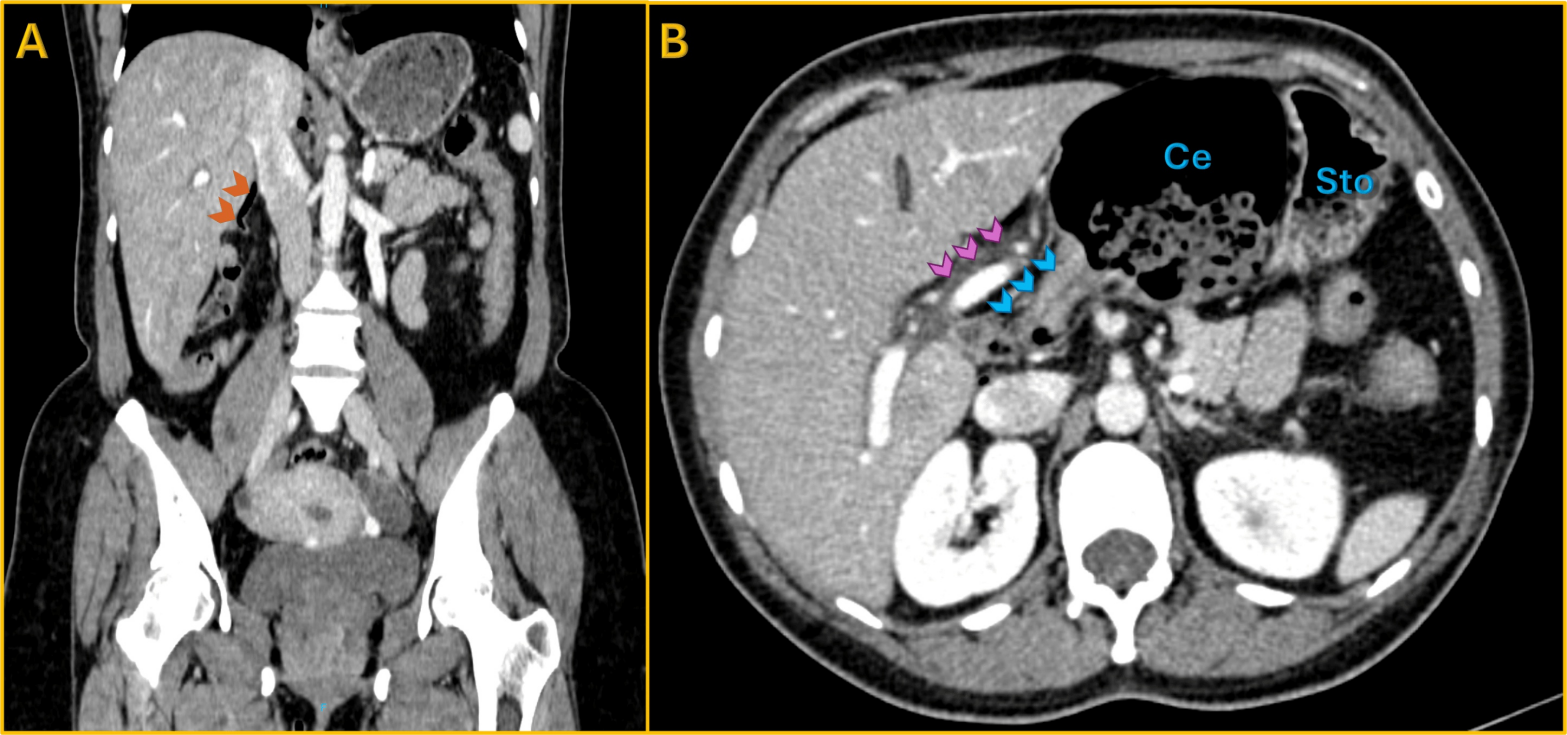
Subhepatic appendix and axial confirmation of cecal herniation behind the portal vein (A) Coronal contrast-enhanced CT showing the appendix in an abnormal subhepatic position (orange arrowheads). (B) Axial CT image demonstrating the main portal vein (purple arrowheads) displaced anteriorly, with the terminal ileum coursing behind it into the lesser sac (blue arrowheads). The cecum (Ce) is visualized within the lesser sac, medial to the stomach (Sto), confirming the path of herniation through the foramen of Winslow.
